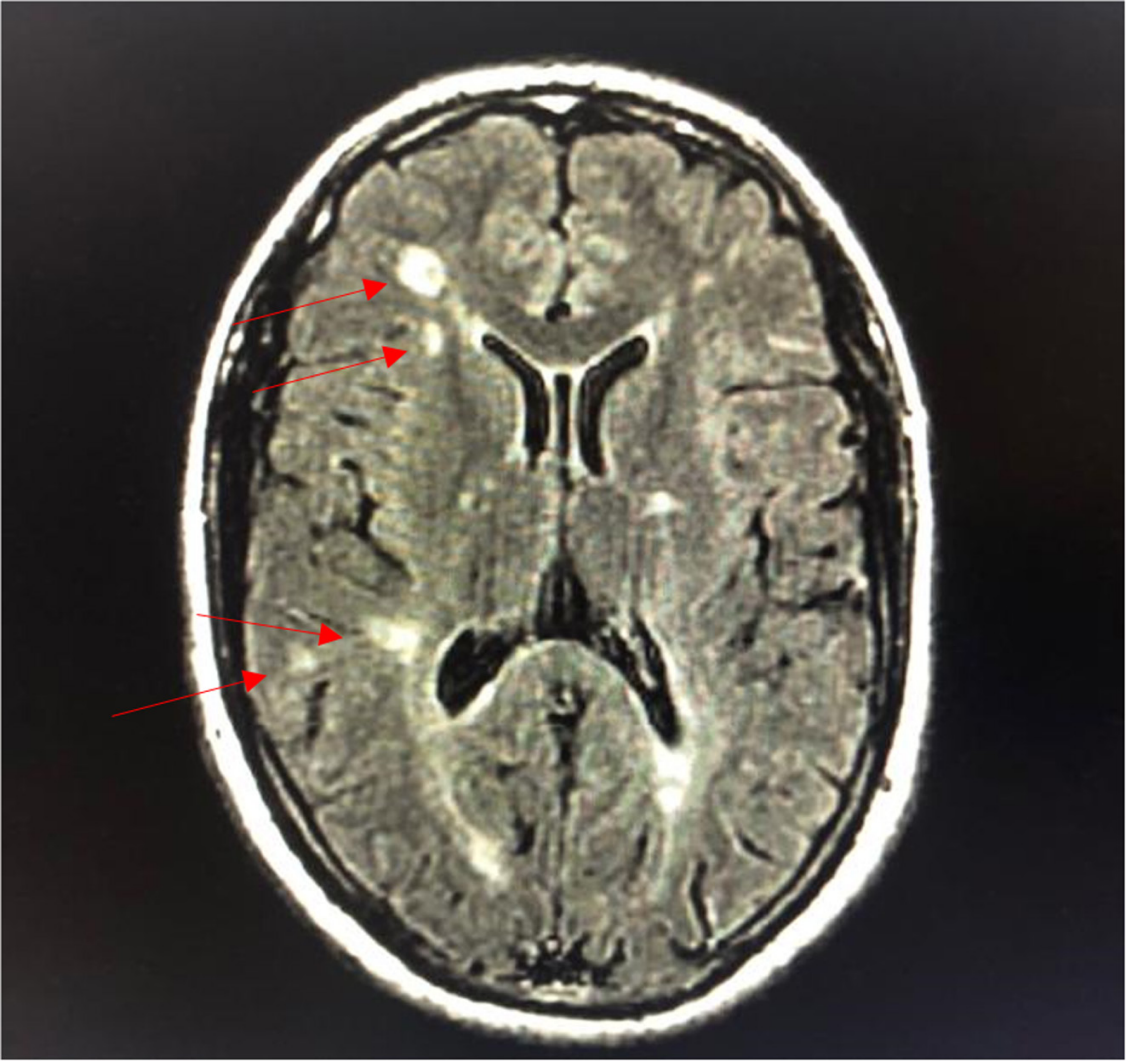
T2 FLAIR MRI of the brain in August year X showing signal abnormalities in the subcortical area of the frontal, parietal and temporal lobes, as well as the periventricular white matter.
